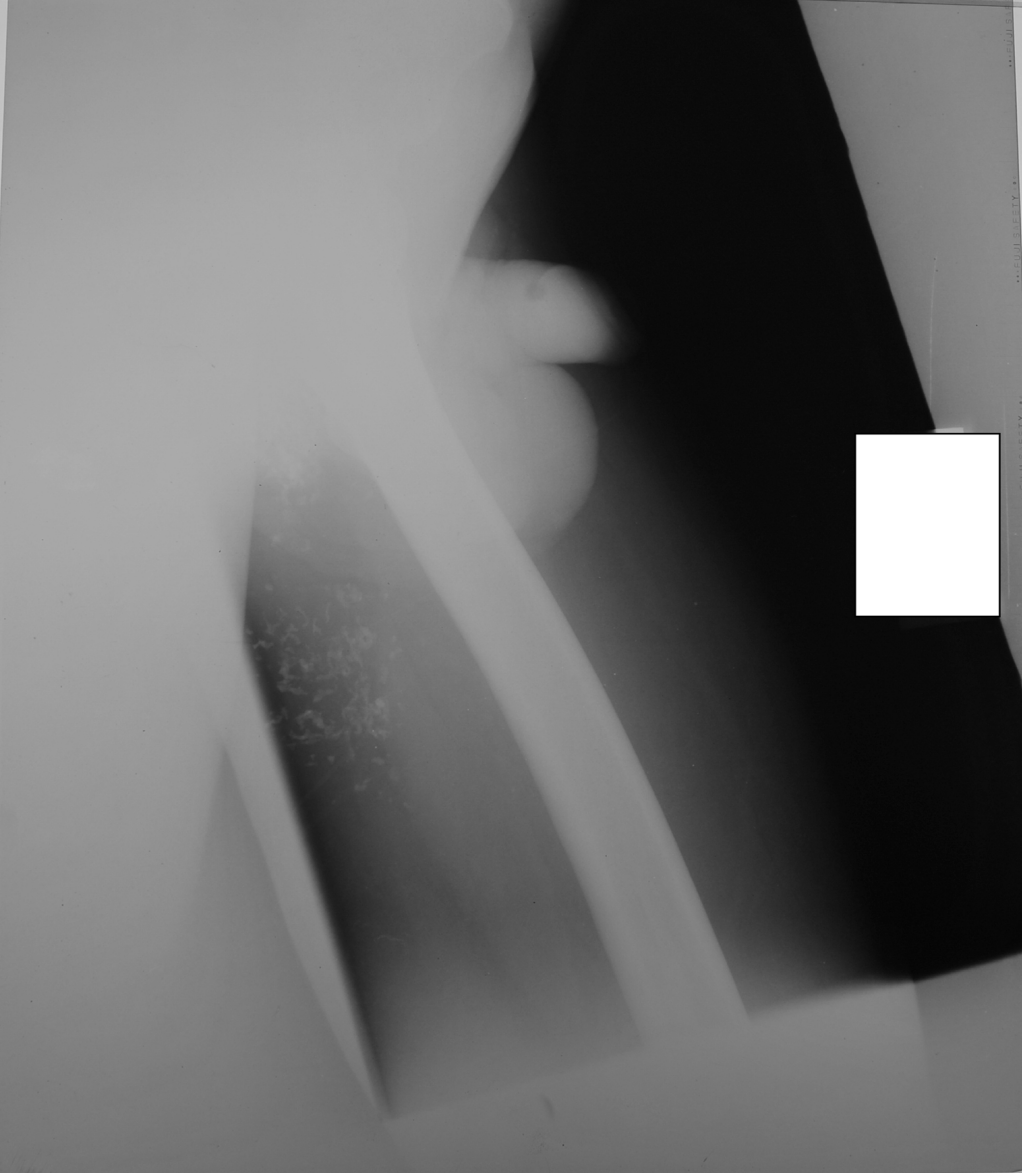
Roentgenogram of the left hip and thigh of our patient. Calcifications that might be the sequelae of a healed tuberculous abscess can be seen.
